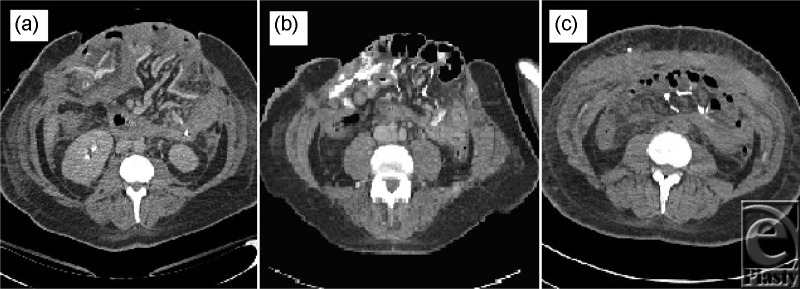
(*a*) NHS hospital admission CT abdomen, note open laparotomy wound and herniating bowel. (*b*) CT scan 6 months after initial injury shows herniated bowel, retraction of the abdominal musculature and incidental finding of diffuse peritoneal calcifications prior to components separation. (*c*) Post components separation closure CT, note remnant calcifications. CT indicates computed tomography.
